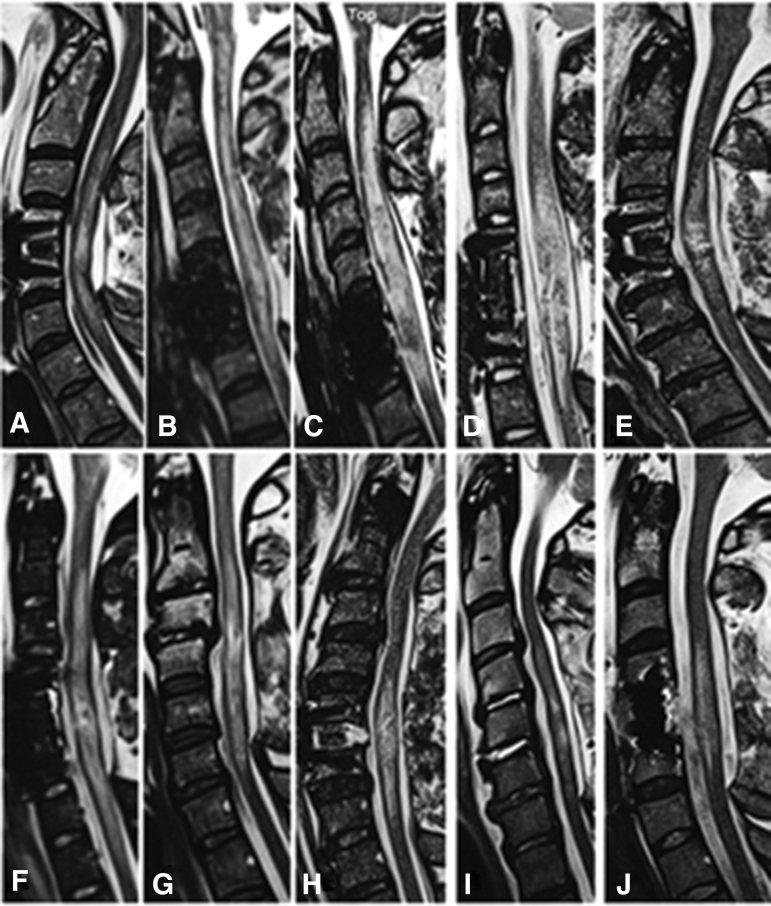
Post-operative midsagittal magnetic resonance imaging (MRI) views from 10 randomly selected patients with adequate decompression plates (A–J). Adequate decompression was defined as presence of cerebrospinal fluid (CSF) interface in front, behind, or in front/behind of the spinal cord. In panels A, C, and F, the CSF interface is partially missing behind the spinal cord, which might be because of the effect of gravity while the patient is in the supine position in the MRI gantry.
